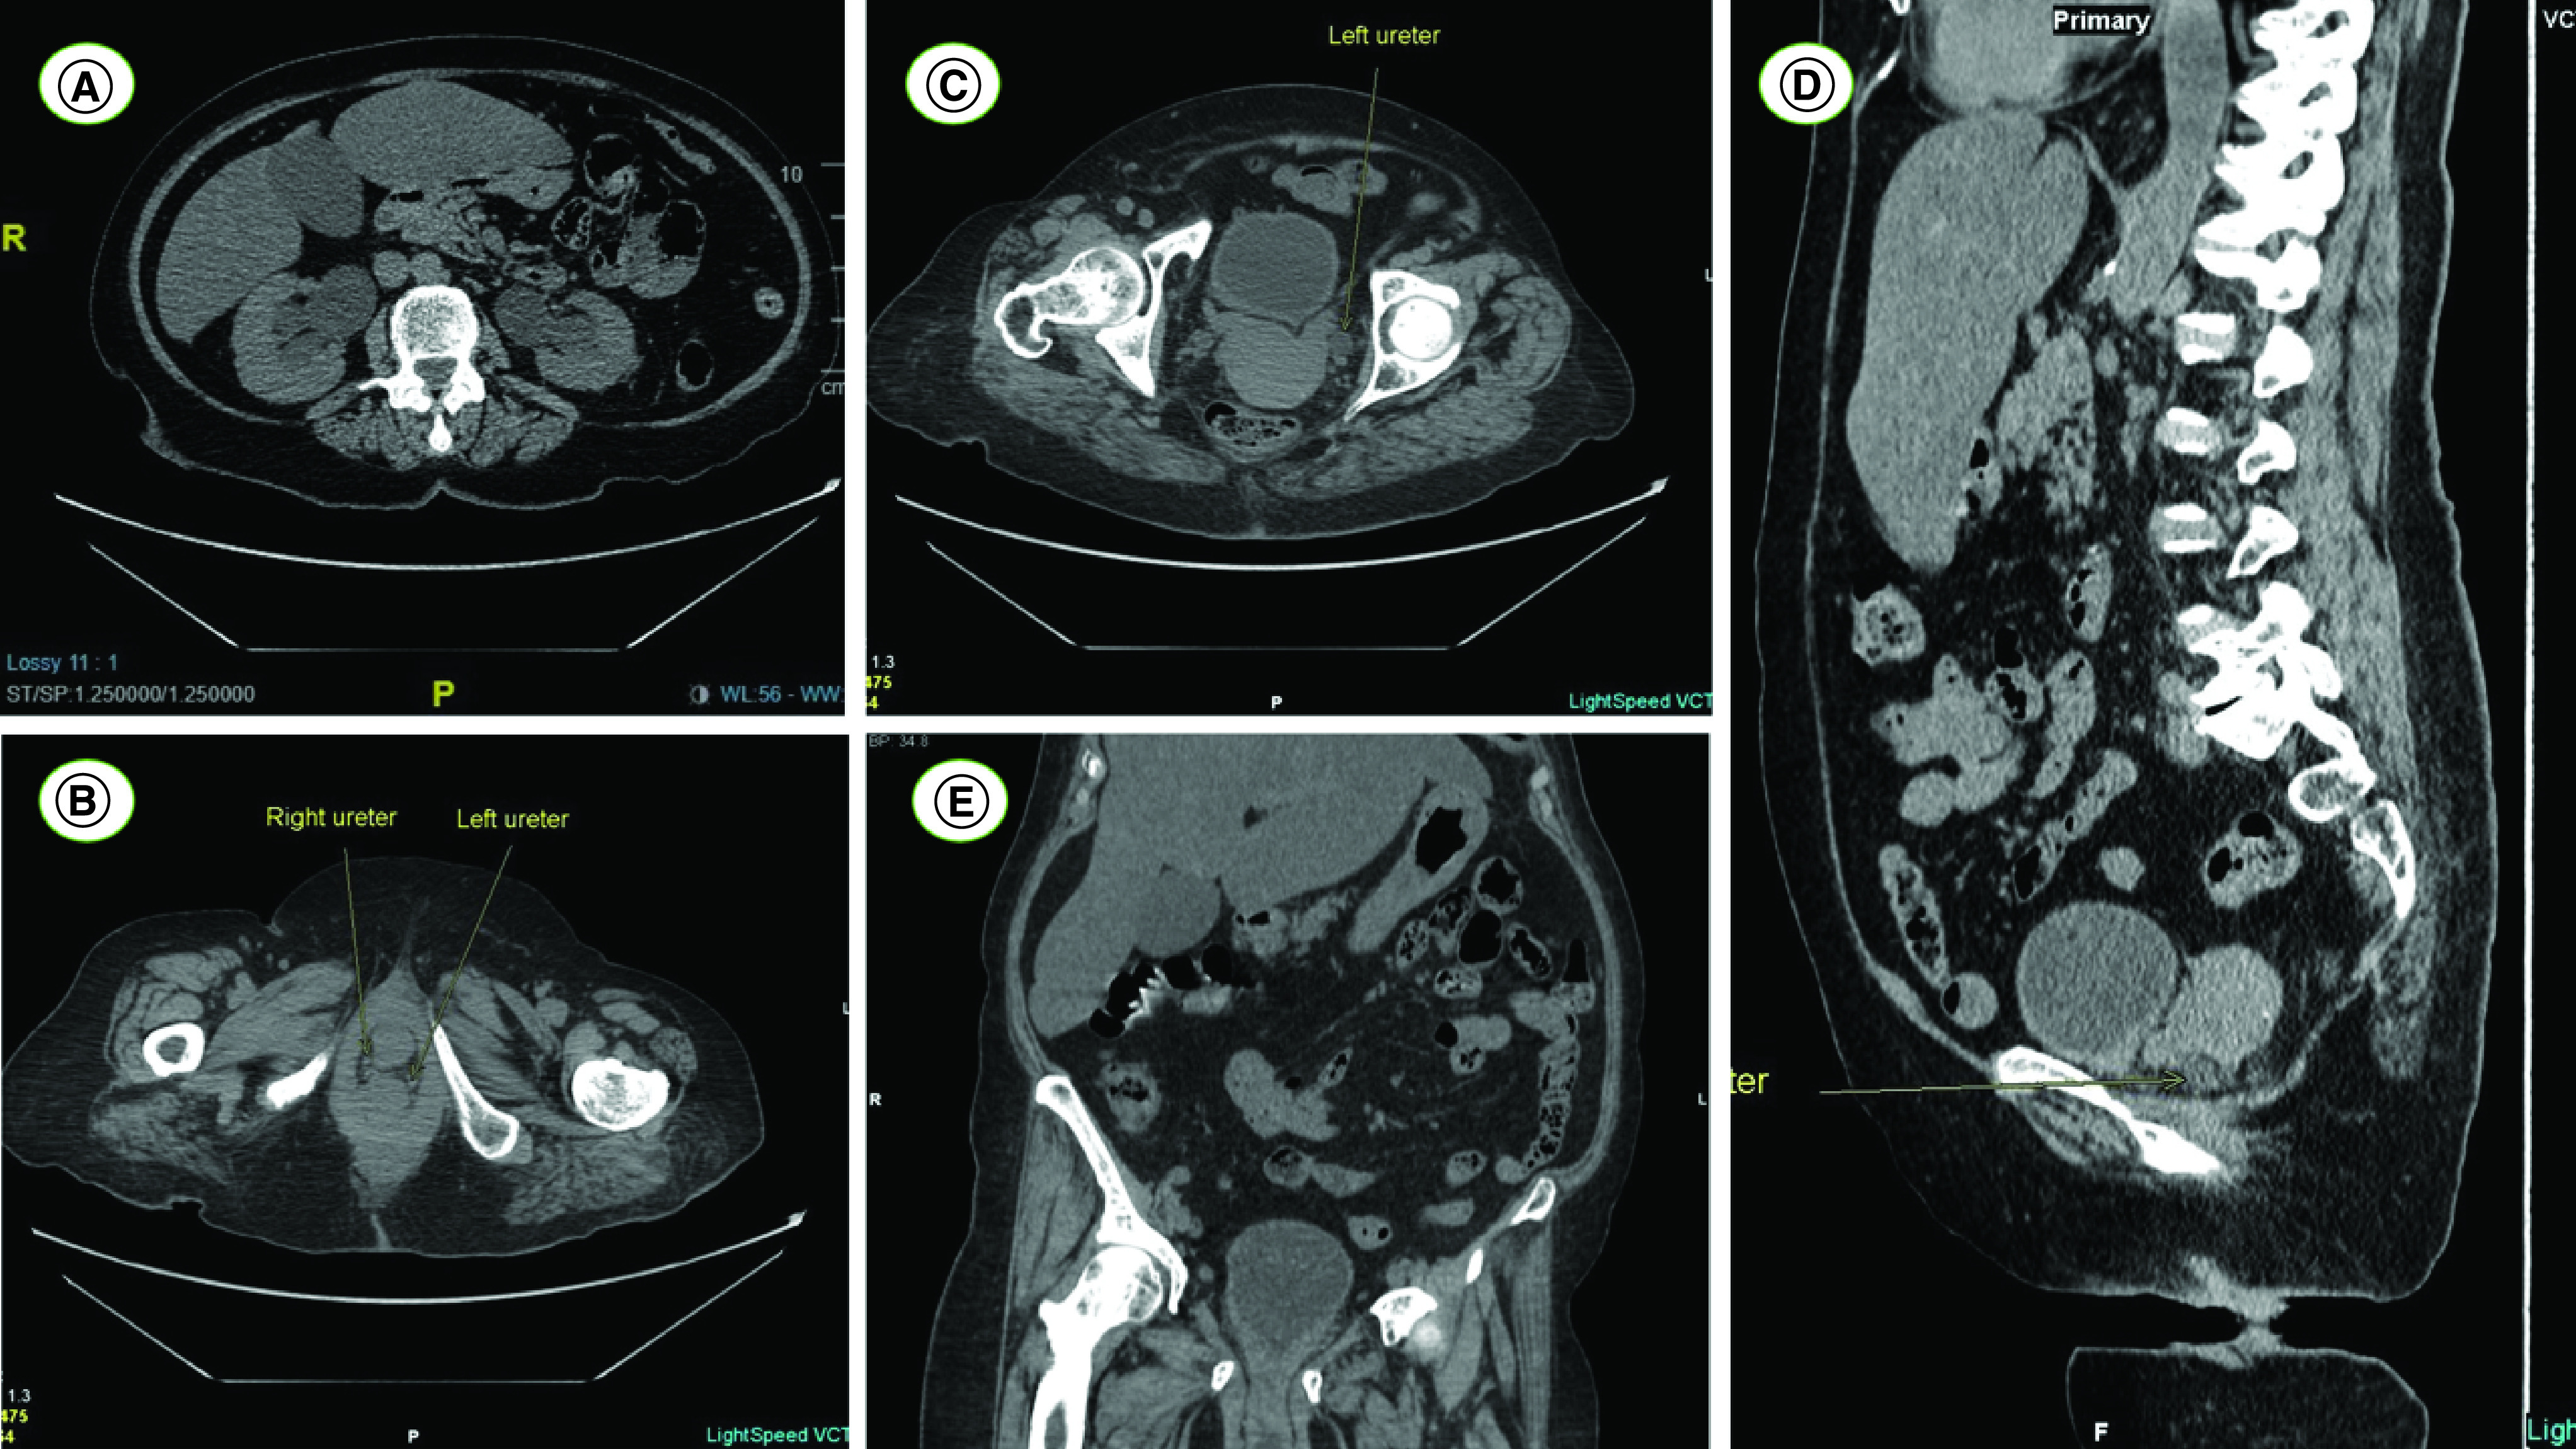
Computed tomography scan of our patient. (A) Computed tomography scan without intravenous contrast showing severe bilateral hydronephrosis; (B) CT scan without intravenous contrast showing bilateral ureteral compression by the urogenital hiatus; (C) CT scan without intravenous contrast showing bilateral hydroureters; (D) CT scan without intravenous contrast showing left ureter compression between uterine fundus and pelvic diaphragm; (E) CT scan without intravenous contrast showing grade 4 cystocele and associated colpocele.
